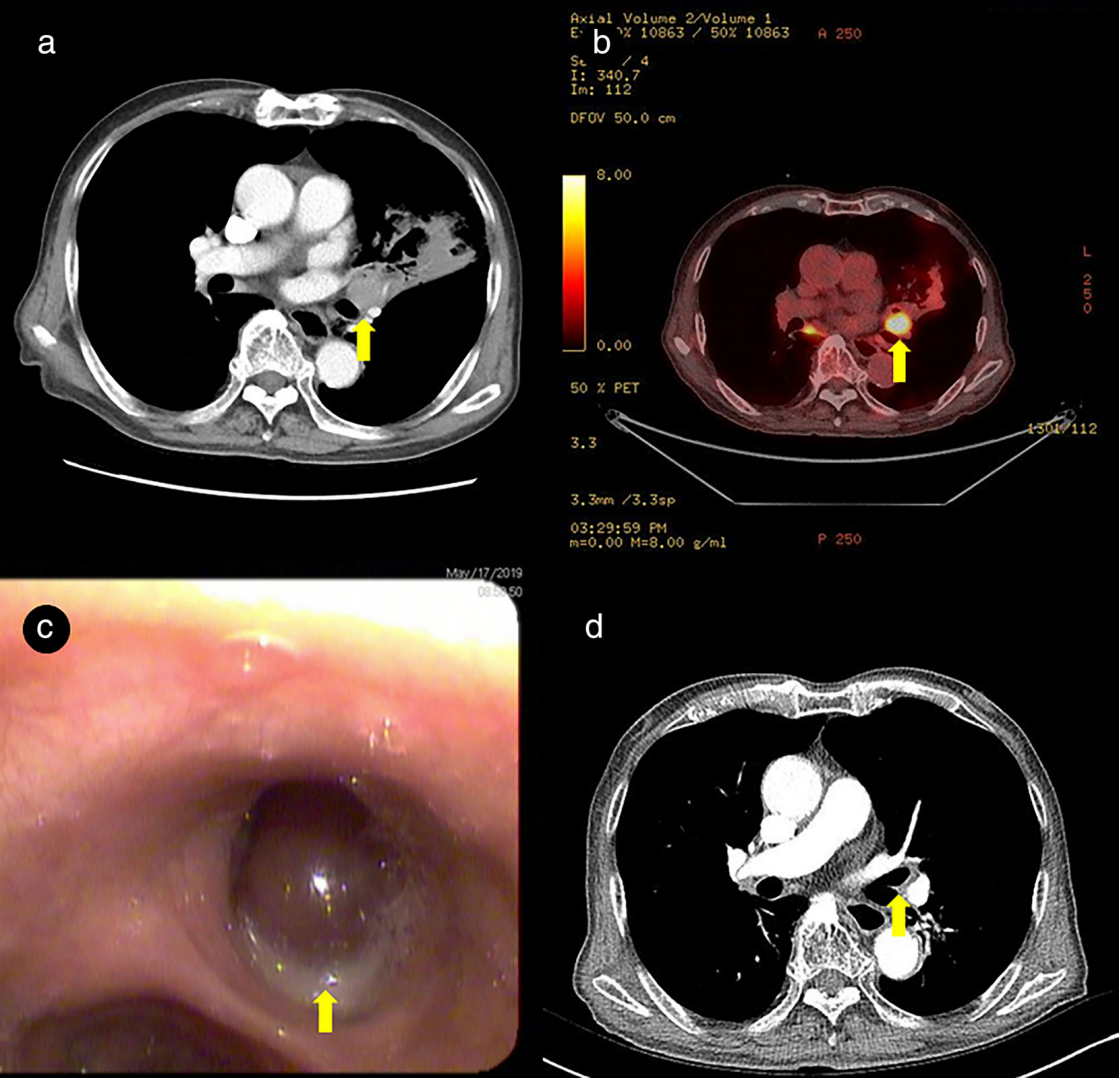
(a) Chest computed tomography (CT) revealed a mass obstructing the left upper lobar bronchus and obstructive pneumonitis. (b) Positron emission tomography (PET) CT revealed a 2.5‐cm fluorodeoxyglucose‐avid nodule involving the left upper lobar bronchus and distal pneumonitis. (c) Fiberoptic bronchoscopy revealed a protruding mass in the left upper lobar bronchus. (d) Chest CT revealed marked improvement of a mass obstructing the left upper lobar bronchus and obstructive pneumonitis after radiotherapy
